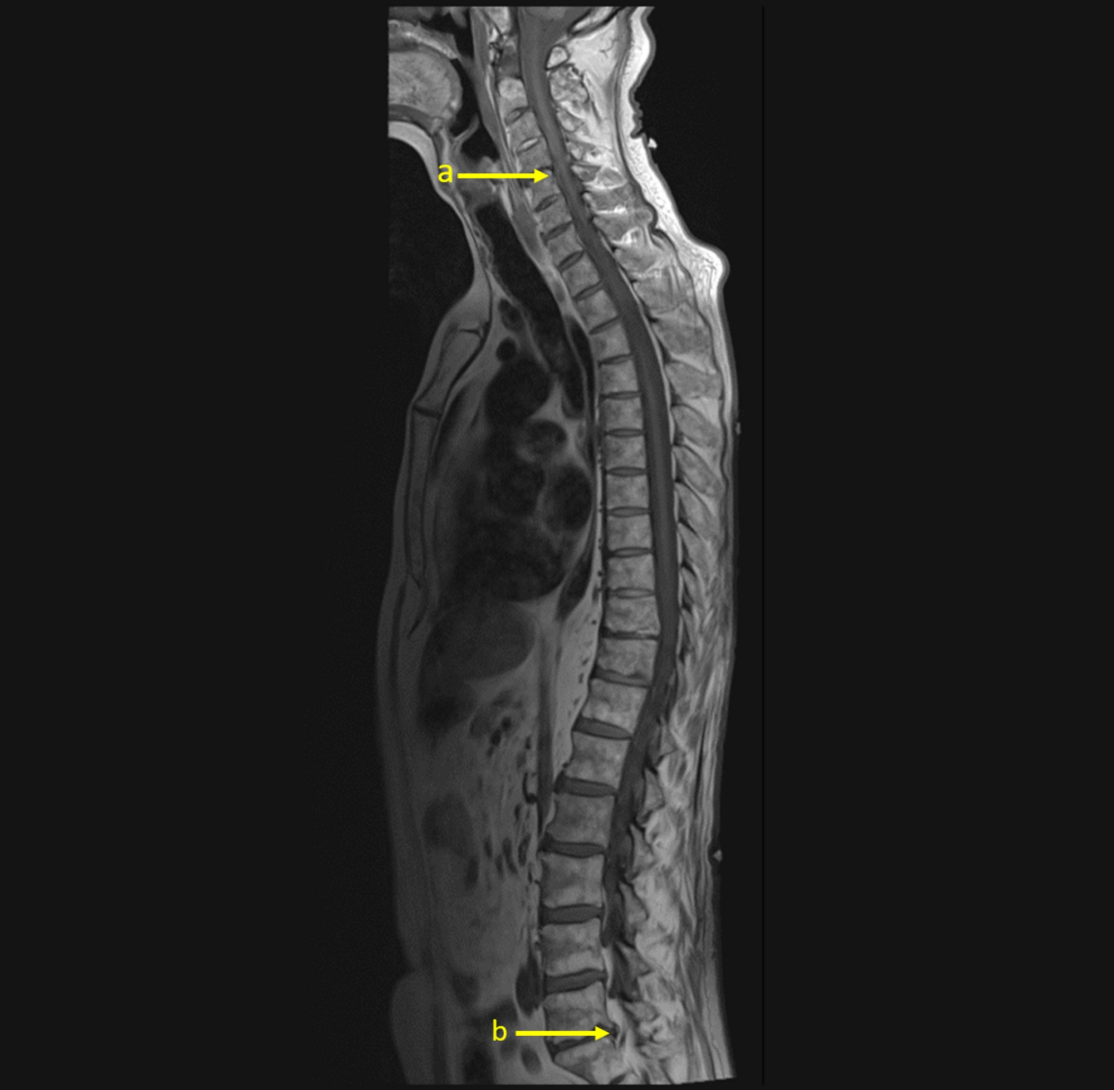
T1-weighted magnetic resonance imaging (MRI) without contrast of whole spine. T1-weighted sagittal MRI without contrast of complete spine demonstrating absence of fracture, subluxation, and abnormal cord signal in cervical, thoracic, and lumbar spine. a) Disc osteophyte complex present at C4-C5 contacting thecal sac with probable mild-moderate spinal canal stenosis. b) Moderate bilateral neural foraminal narrowing at L5-S1 present.
